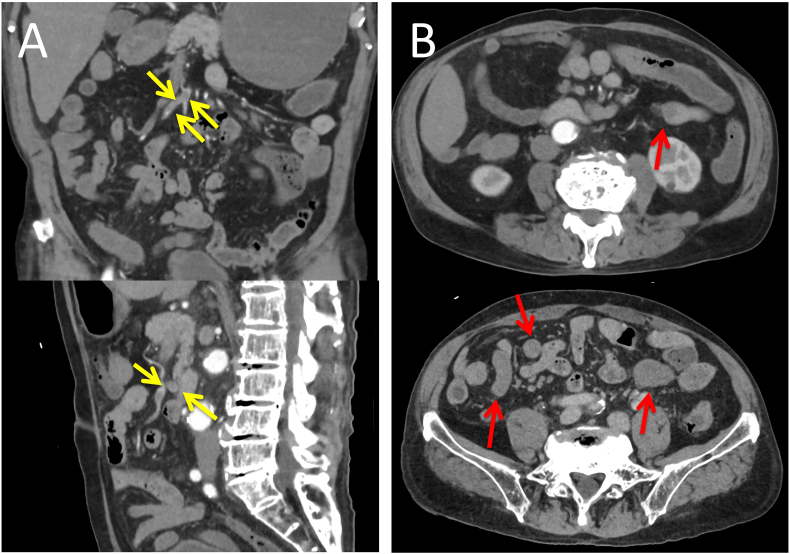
Contrasted-enhanced CT A: Occlusion of the SMA associated with a thrombus is noted. B: Contrast enhancement on the small intestine is sparse.
